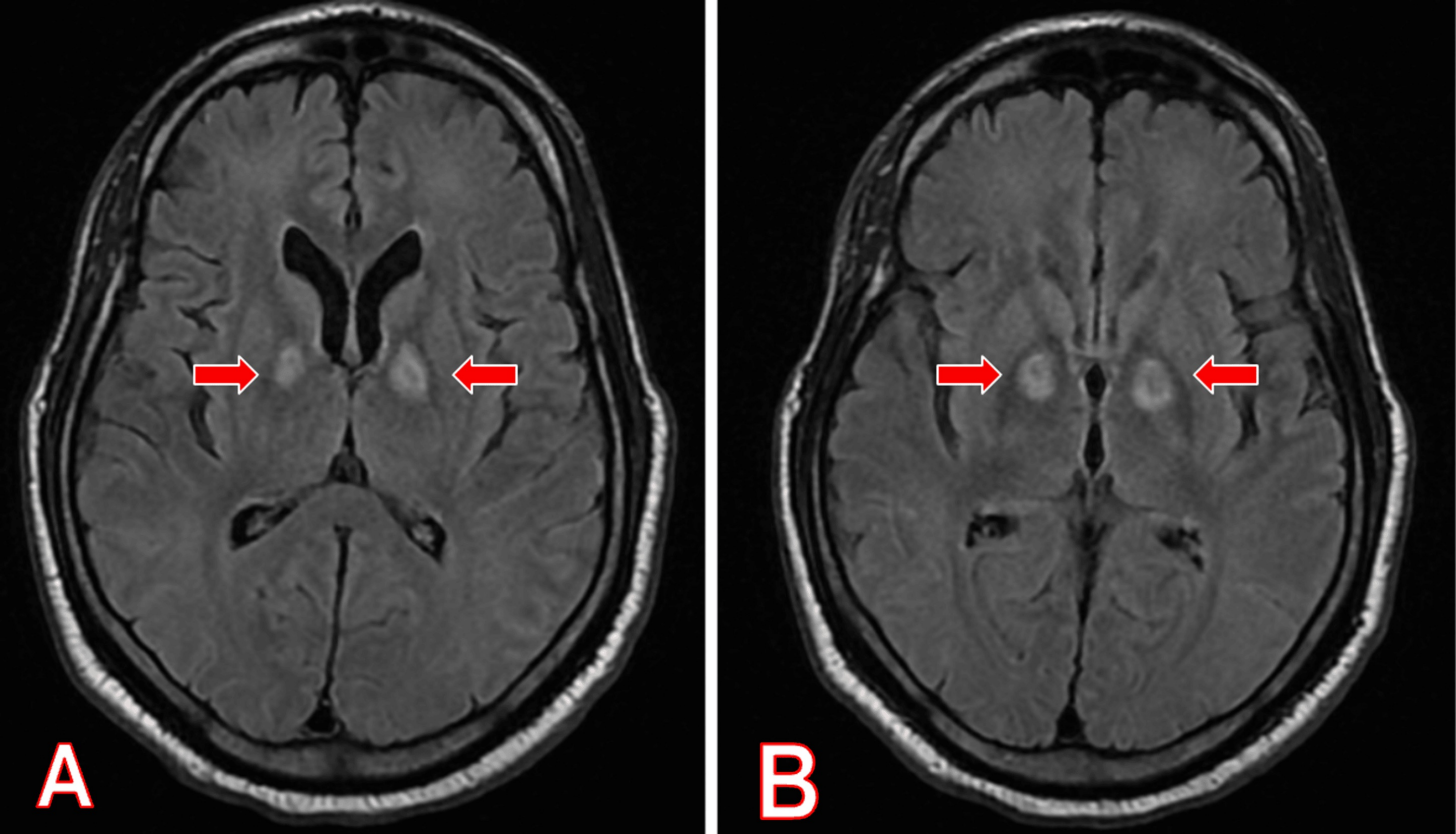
MRI brain without contrast, T2 FLAIR sequence, axial view, demonstrating bilateral basal ganglia infarction. Red arrows show bilateral basal ganglia infarctions on MRI brain without contrast, T2 FLAIR sequence on axial view. (A) First series. (B) Second series. FLAIR: fluid-attenuated inversion recovery
